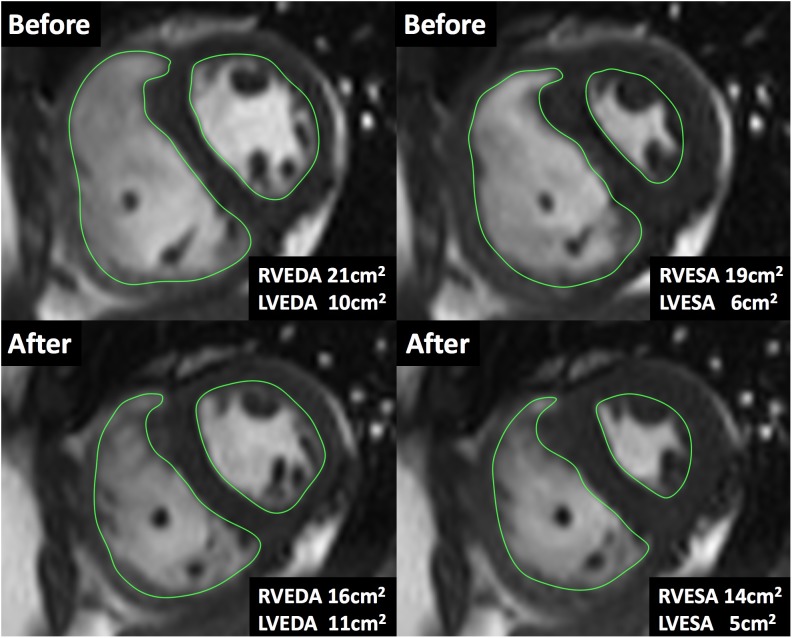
Cardiac magnetic resonance imaging during acute iloprost inhalation. A case of the short-axis view by CMR cine. The areas were measured at the same location before and after iloprost inhalation. After iloprost inhalation, RV end-diastolic area (RVEDA) was reduced from 21 to 16 cm^2^ and RV end-systolic area (RVESA) was reduced from 19 to 14 cm^2^.
